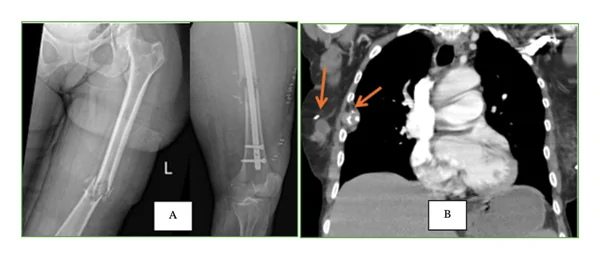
(A) X‐ray of the left femur midshaft diaphyseal compound fracture pre‐ and postsurgery. (B) Computed tomography of the chest with an expansile lytic lesion of the right 5th rib and a right breast mass.
